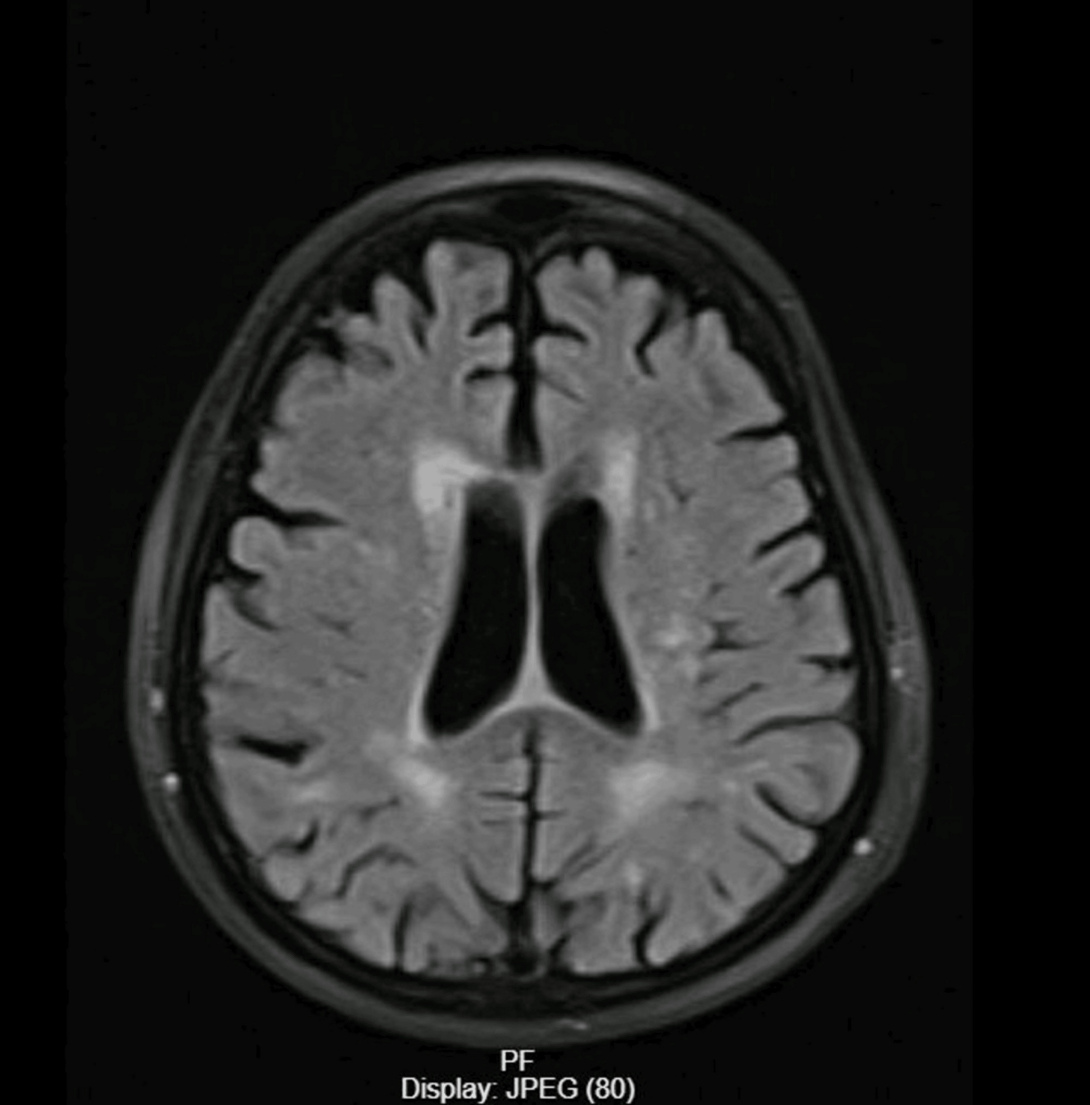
MRI brain The image shows dilated cerebral ventricles with no midline shift or deformity, bilateral periventricular deep and subcortical white matter, and patchy areas of high FLAIR WI signals. The picture is impressive of chronic arteriolosclerotic leukoencephalopathy. FLAIR: fluid-attenuated inversion recovery; WI: weighted image
